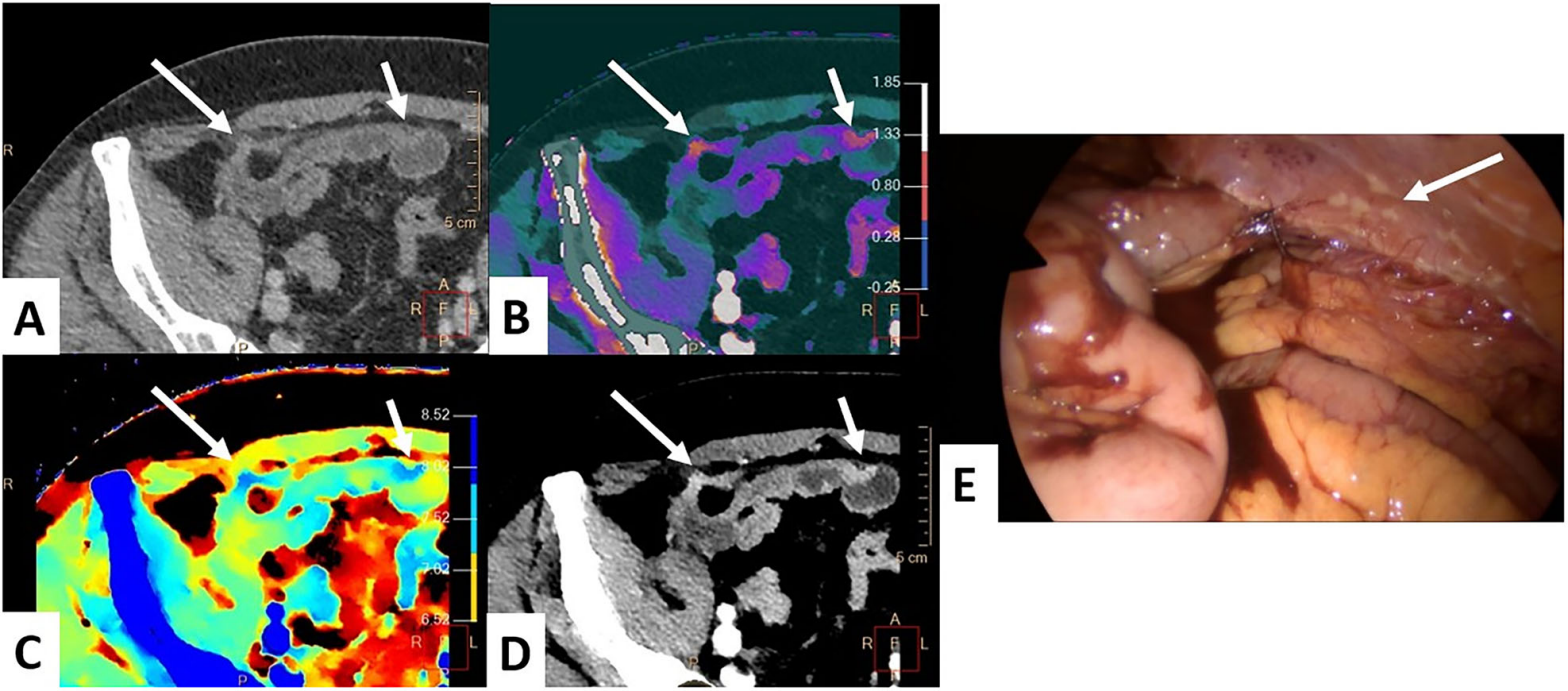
61-year-old man scheduled for CRS and HIPEC for a transverse colon adenocarcinoma with synchronous PM. DLCT (Iqon, Philips) in axial section at VP. A The conventional CT image shows a barely visible nodular thickening of the parietal peritoneum in the right iliac fossa (long arrow) and the small-bowel serosal nodule (short arrow). B–D The iodine map (B), Zeff map (C), and 40-keV VMI image (D) show much better the detection of the nodular thickening (long arrow), compared with the adjacent abdominal wall and small-bowel loops, as well as the small-bowel serosal nodule (short arrow). E Laparoscopy confirms both peritoneal metastases, including the parietal peritoneal nodule (long arrow). CRS, cytoreductive surgery; CT, computed tomography; DLCT, dual-layer computed tomography; HIPEC, hyperthermic intraperitoneal chemotherapy; PM, peritoneal metastases; Zeff, Z-effective
